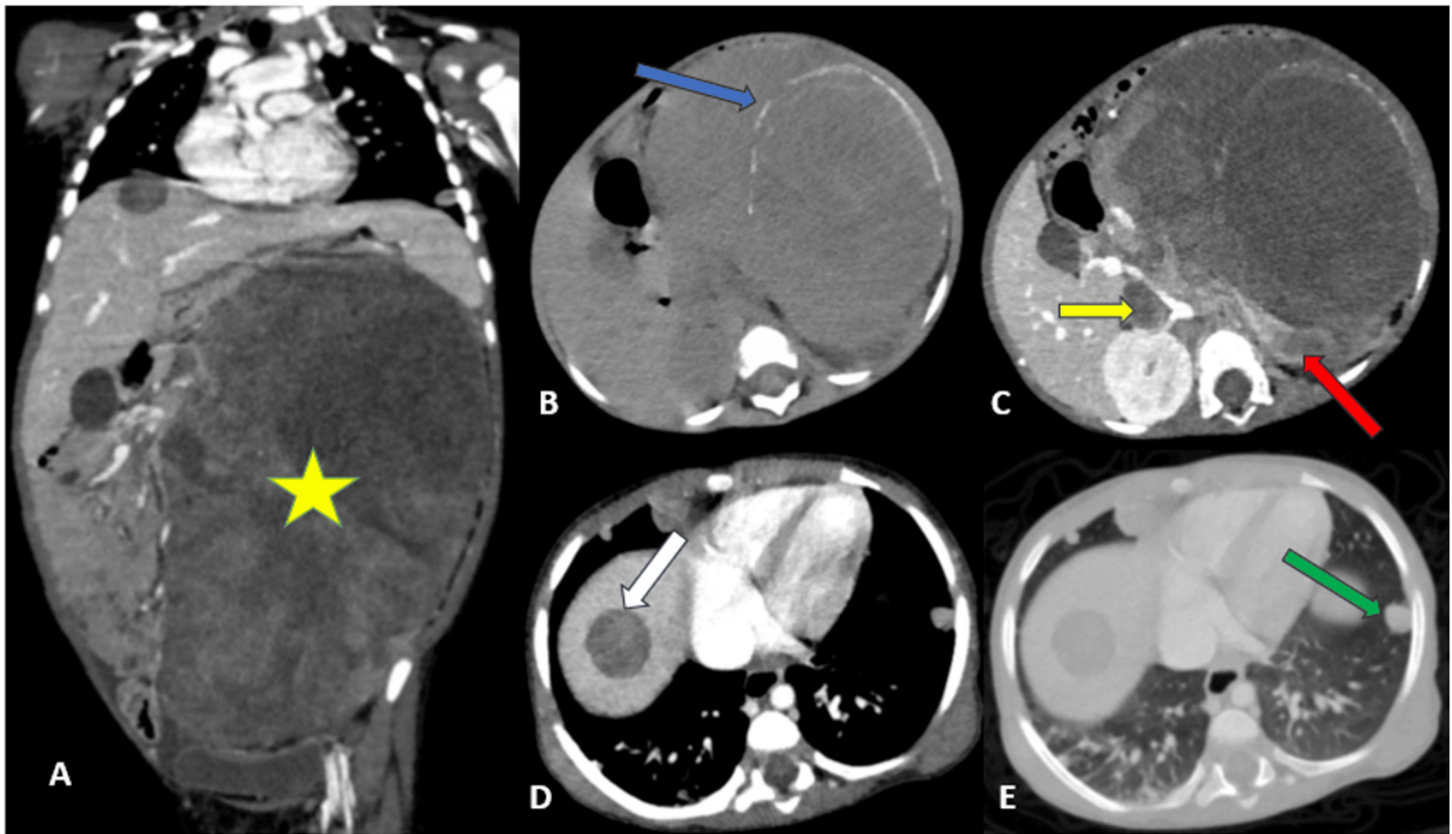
Thoraco-abdominopelvic CT scan with (A, C, D, E) and without (B) contrast enhancement, shown in coronal reconstruction (A) and axial sections (B, C, D, E). The images demonstrate a large left-sided retroperitoneal mass (asterisk) exhibiting the spur sign with the left kidney (red arrow), containing internal calcifications (blue arrow), and showing heterogeneous, low enhancement. The scan also reveals an intravascular tumor thrombus within the IVC (yellow arrow), as well as pulmonary (green arrow) and hepatic metastases (white arrow). IVC, inferior vena cava
